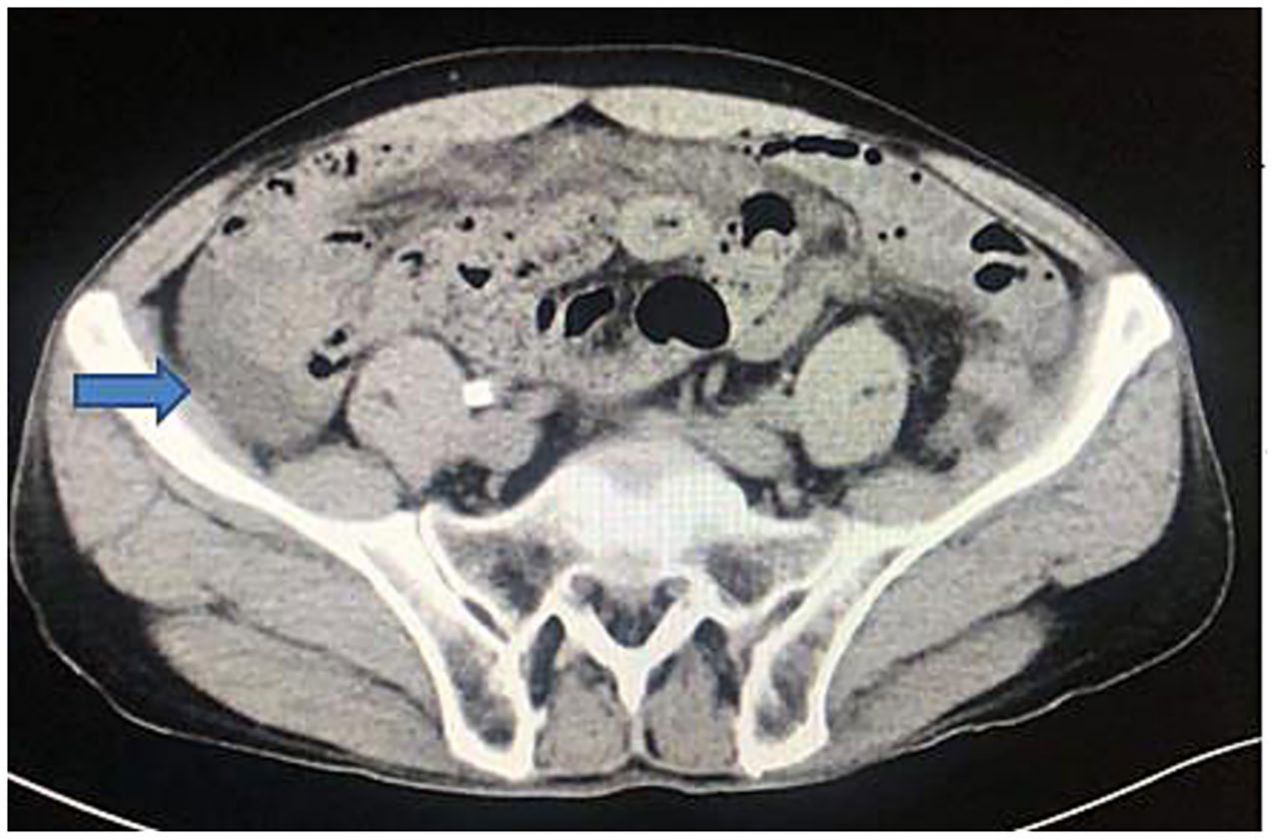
Abdominal computed tomography showing abdominal fluid.
